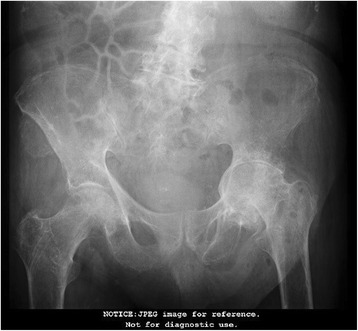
Anteroposterior X-ray of the pelvis. Multiple lytic lesions in the femur and humeral head
